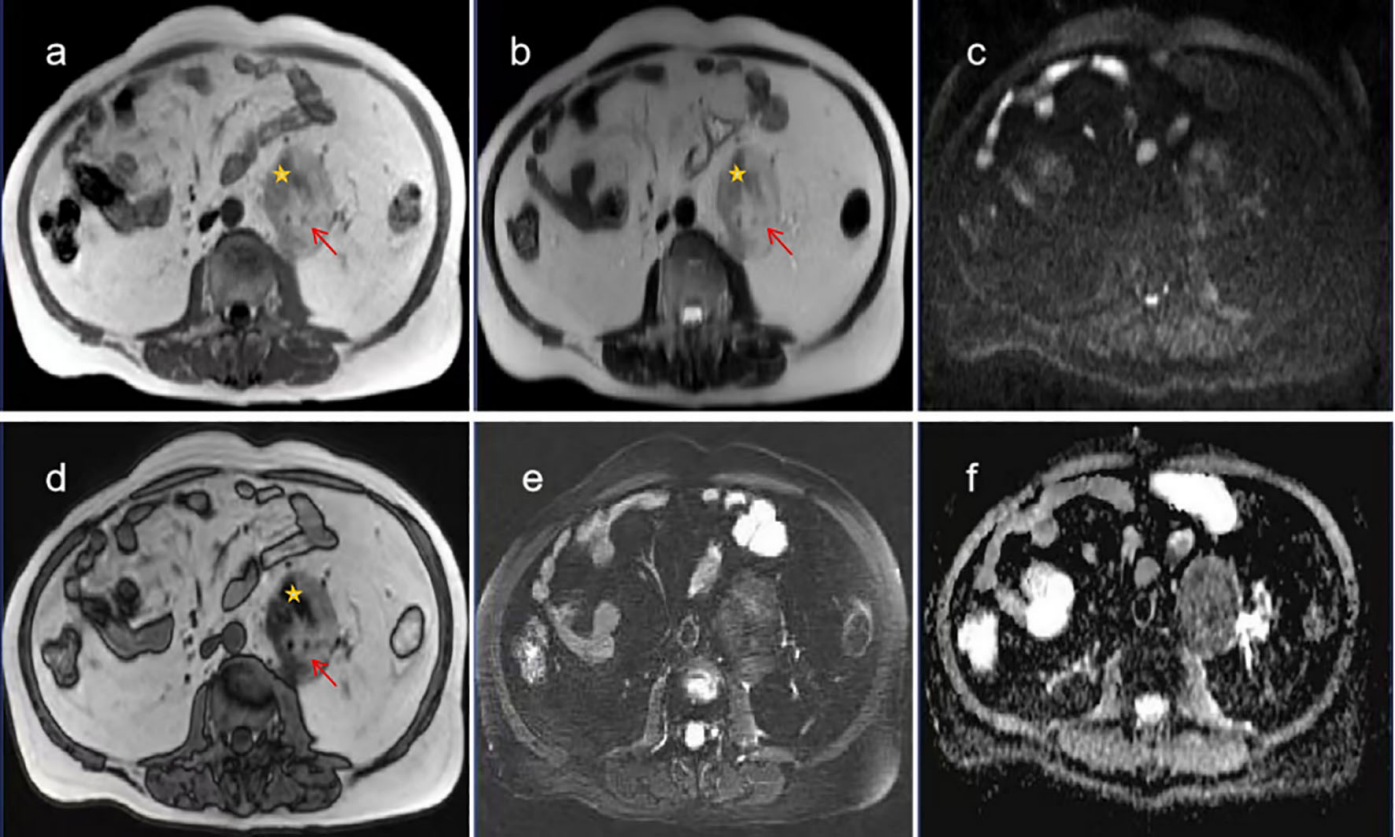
MRI images of extra-adrenal retroperitoneal myelolipoma. (a–d) Lipid components decrease in the reverse phase. (b, e) Mature fat decreases in the T2W1 lipid compression sequence. (c, f) DWI and ADC sequences show mild diffusion restriction in some components of the mass (Mature adipose tissue, Lipid-containing myeloid tissue).
